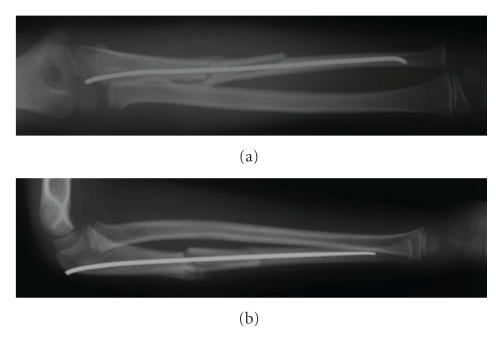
Anteroposterior (a) and lateral (b) radiographs of right forearm two weeks after exploration.
